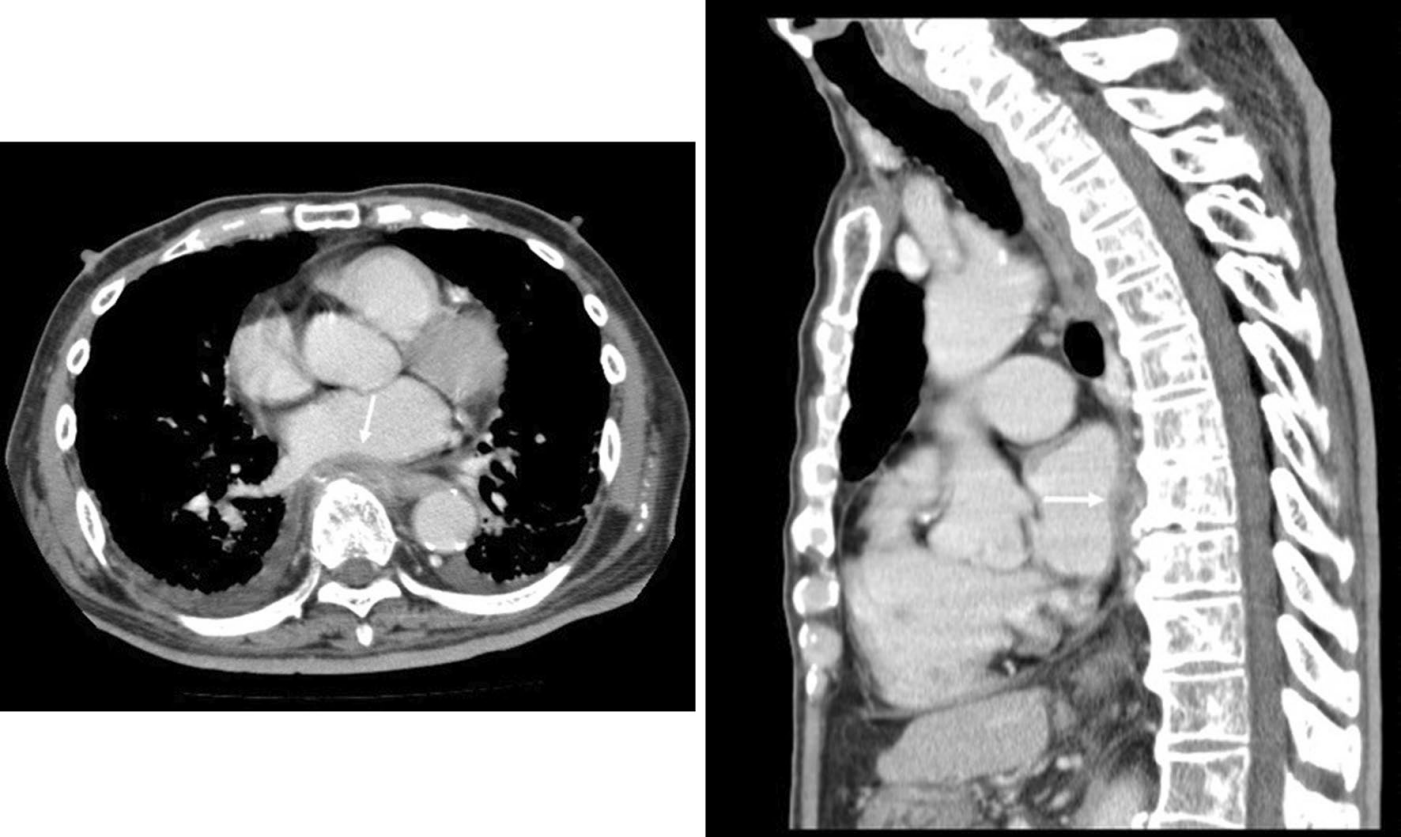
Computed tomography (CT) findings of discitis. The scan shows a soft tissue shadow in front of the vertebral body extending from the 8th/9th intervertebral space (arrows)
